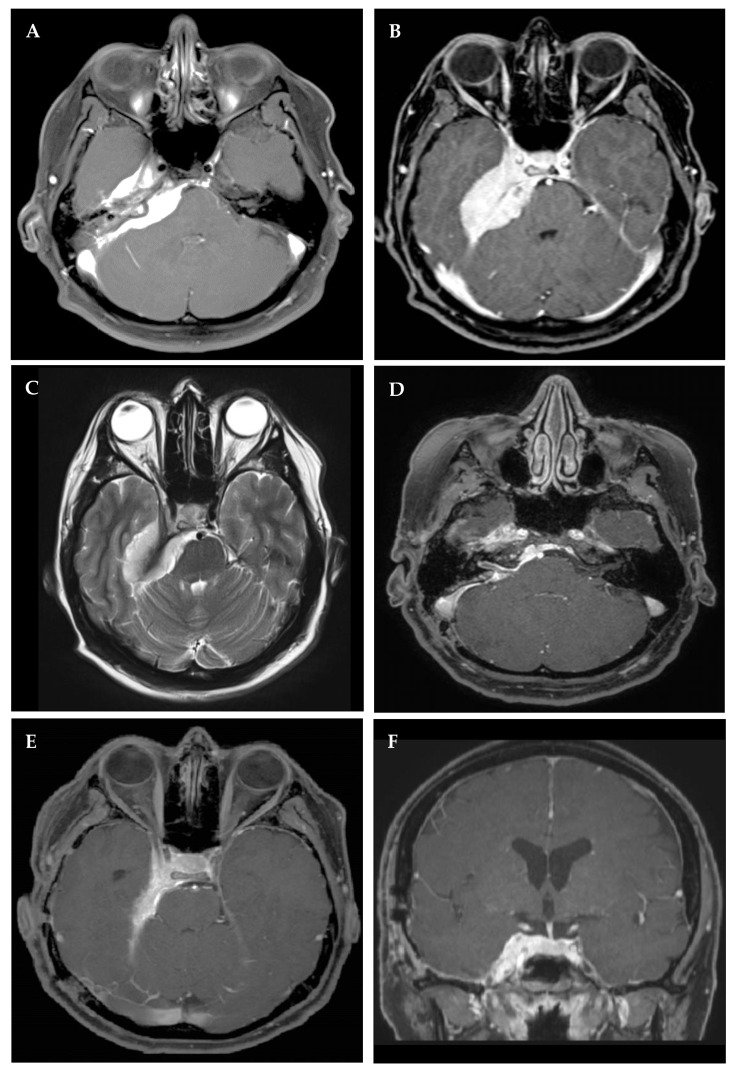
Right petroclival meningioma in a 48-year-old female who presented with tinnitus, diplopia and vertigo, undergoing a staged resection by (1) retrosigmoid and (2) pterional craniotomy. Preoperative magnetic resonance imaging (MRI) in axial contrast-enhanced T1 (A,B) and T2 (C) I sequences demonstrating compression of the brain stem and invasion of the cavernous sinus. Residual tumor within cavernous sinus and tentorial edge on postoperative MRI (D–F). A transient palsy of the 4th nerve was noted after the second procedure, resolving fully on follow-up.
